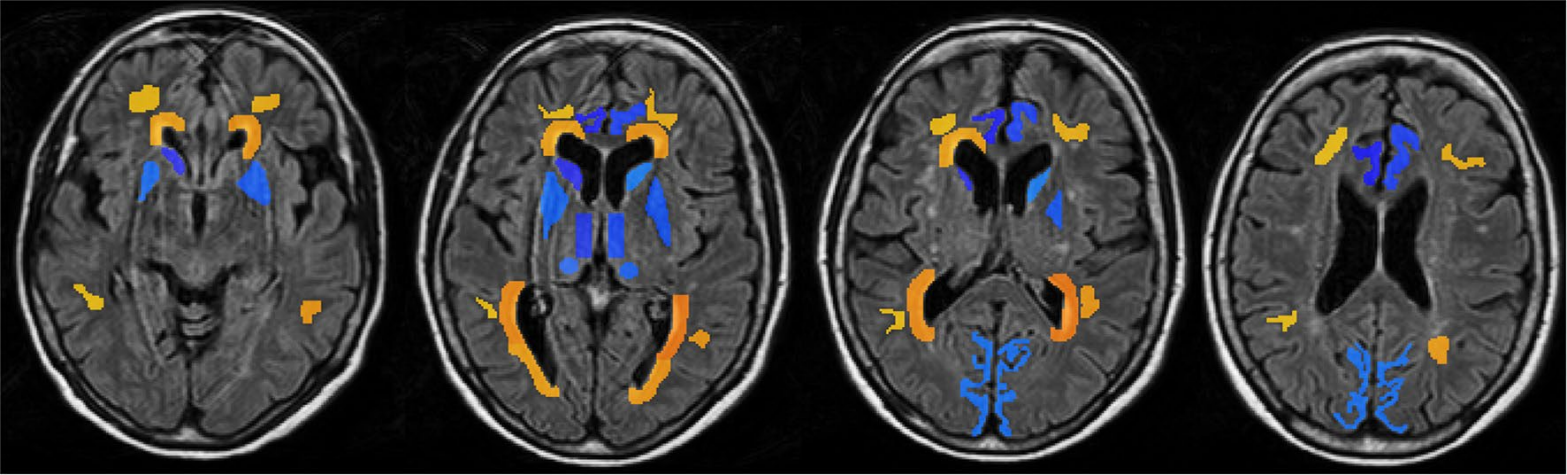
A subset of four slices of FLAIR MRI data, showing manually delineated ROIs with white matter in nuances of orange (normal appearing, anterior periventricular, and posterior periventricular) and gray matter in nuances of blue (cingulate gyrus, caudate head, lentiform nucleus, central thalamus, periventricular thalamus, and occipital cortex). A more detailed description of delineated ROIs has been published previously [14, 15]
